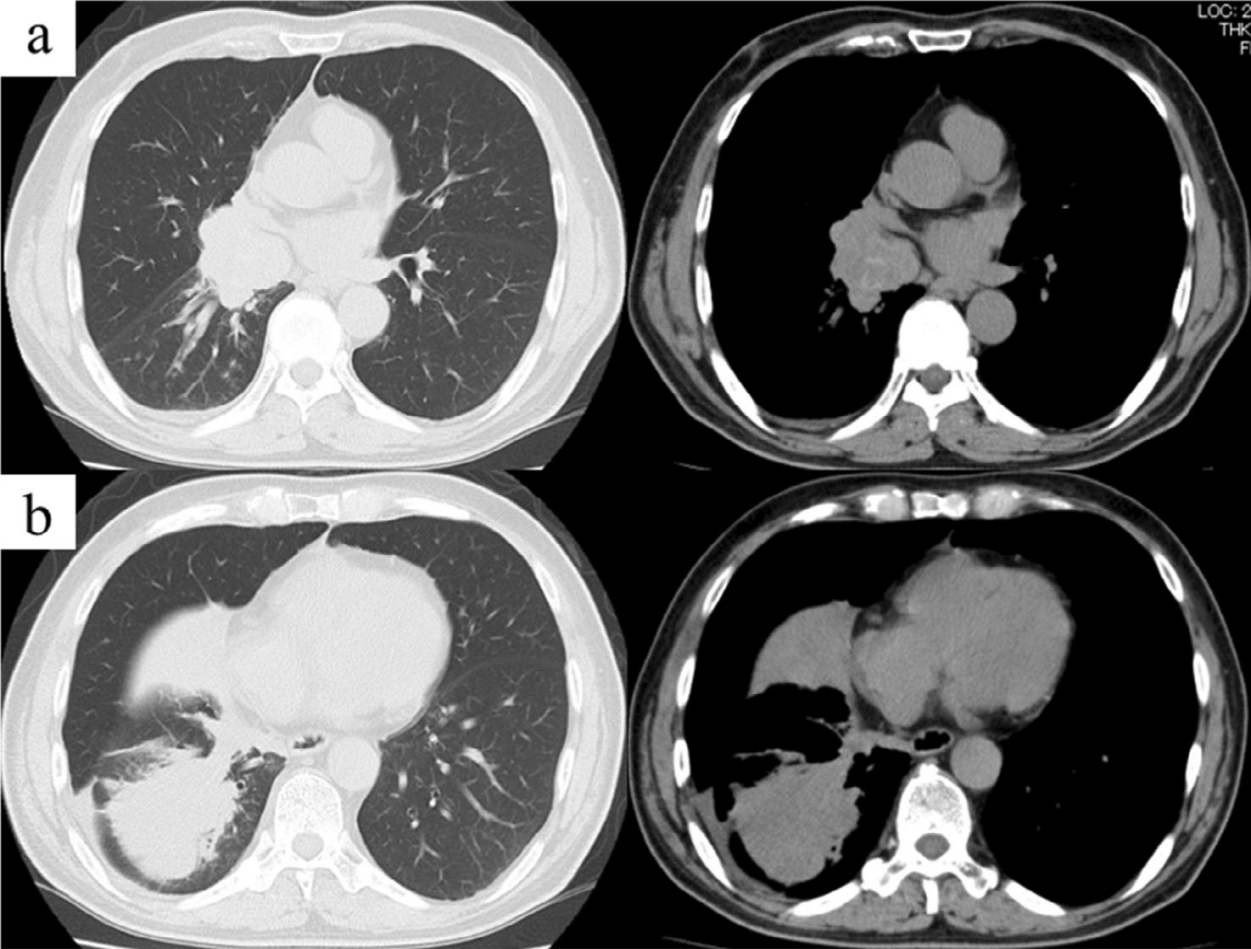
Chest plain computed tomography on the first visit revealed (a) right hilar lymphadenopathy and (b) a mass lesion measuring 70 mm in the right lower lobe.
